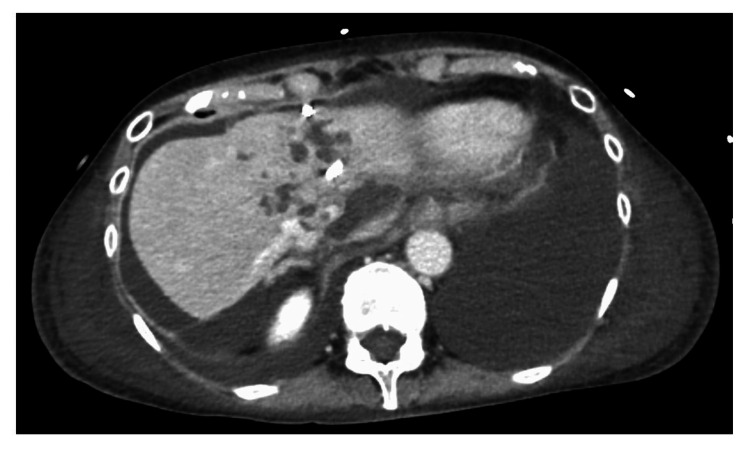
Contrast-enhanced computed tomography images on the 25th day of her illness Contrast-enhanced computed tomography images on the 25th day of her illness revealed that the liver abscess was reduced in size, but the pleural effusion and ascites were increasing.
